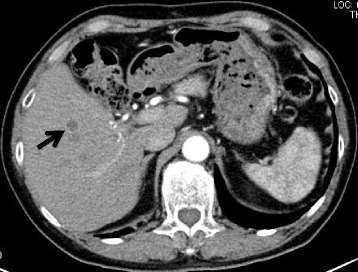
One year after hepatic resection, enhanced computed tomography revealed a solitary, low-density, and hypovascular tumor with a diameter of 10 mm in segment 5 of the liver (*arrow*)
